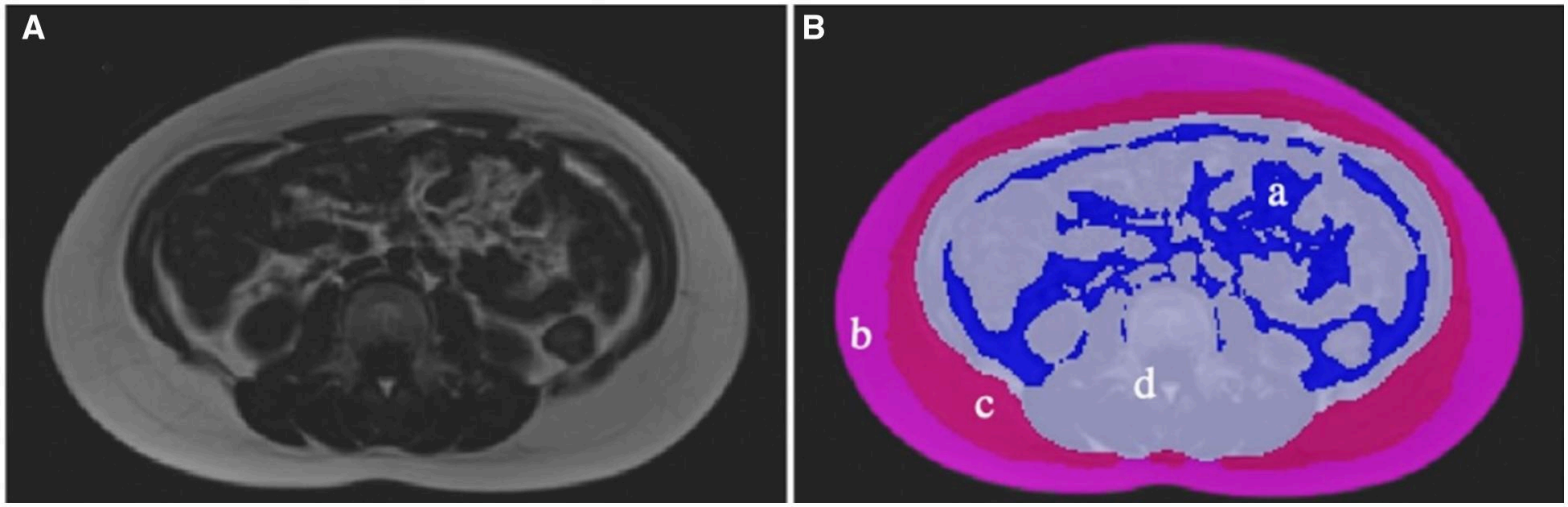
Cross-sectional abdominal MRI for adipose tissue quantification (level L_3_). The participant was a 9-year-old girl with BMI of 25.2. (A) Fat-only Dixon MRI at the level of L_3_. (B) Quantifiable adipose tissue based on Image A at the level of L_3_; a (blue) visceral adipose tissue; b (light pink) superficial subcutaneous adipose tissue; c (dark pink) deep subcutaneous adipose tissue; d (grey) non-adipose tissue. L_3_, lumbar spine level L_3_.
